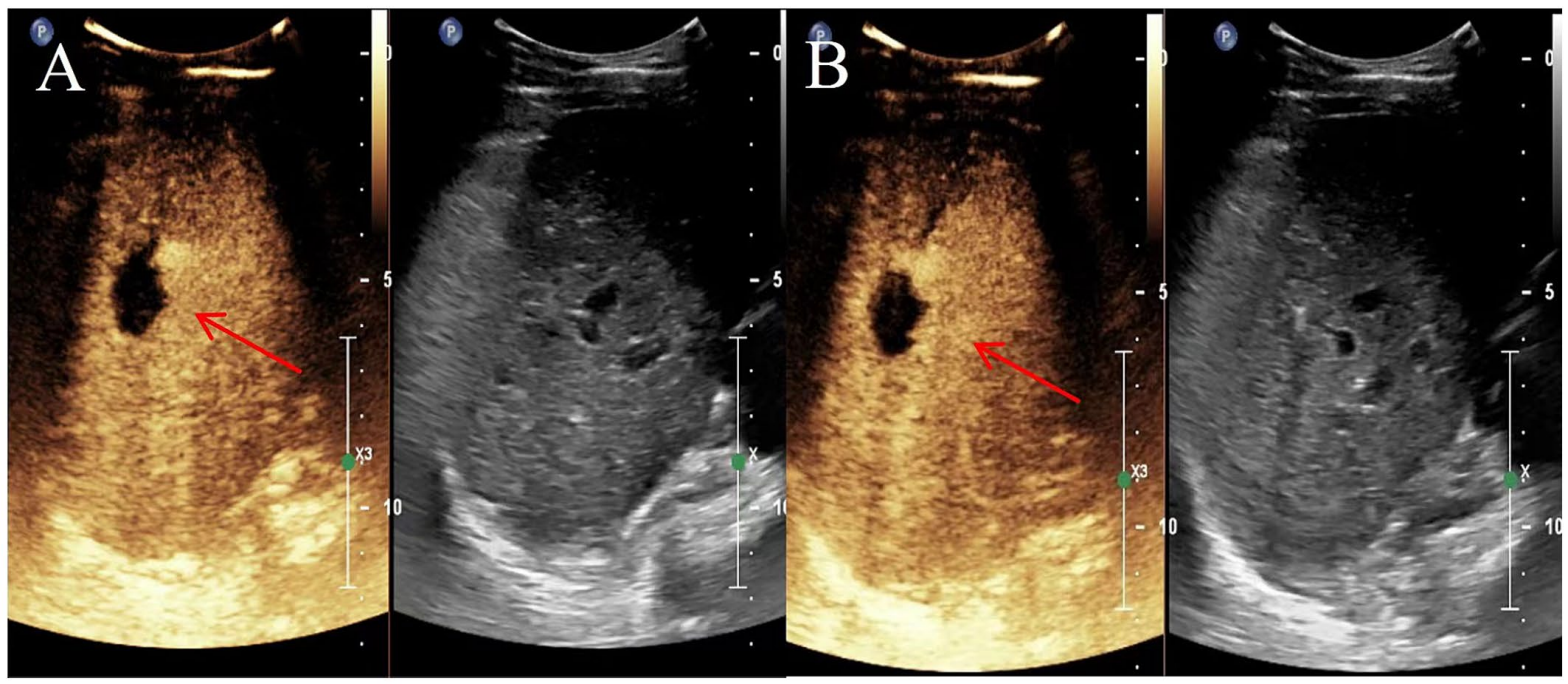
Representative images depicting lesions 6 and 12 months after microwave ablation (MWA) in a 40-year-old female with hepatoalveolar echinococcosis (HAE). (A) Contrast-enhanced ultrasound 6 months after ablation; (B) Contrast-enhanced ultrasound image 12 months after ablation. The red arrow indicates the lesion site.
